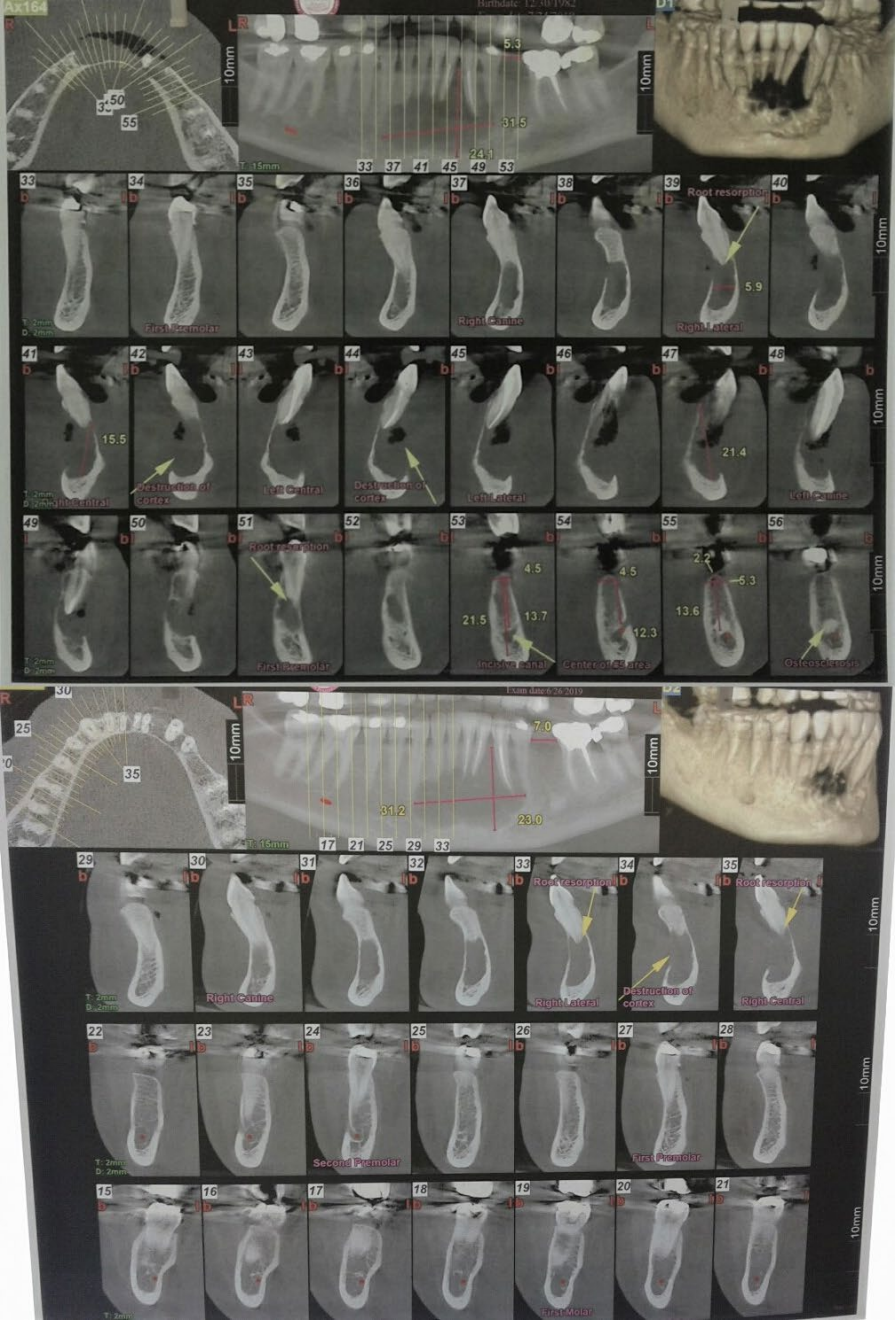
Cone beam computed tomography (CBCT) of the anterior mandible revealing the extent of the lesion. Notice the evident buccal cortex destruction
